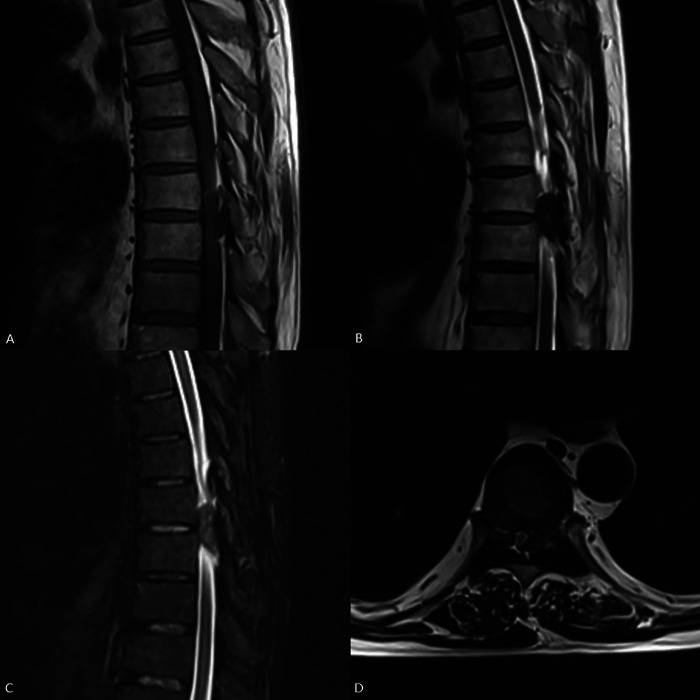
Thoracic spinal epidural lesion on MRI: (A) T1-weighted imaging (T1WI), (B) T2-weighted imaging (T2WI), (C) T2-weighted fat-suppressed imaging (T2W-FS), (D) T2WI transverse axis view. An irregular lesion exhibiting long T1 and short T2 signal intensity is observed in the epidural space of the thoracic spinal canal at the T8-T9 levels. The lesion demonstrated isointensity on T2W-FS. Spinal cord compression is evident.
